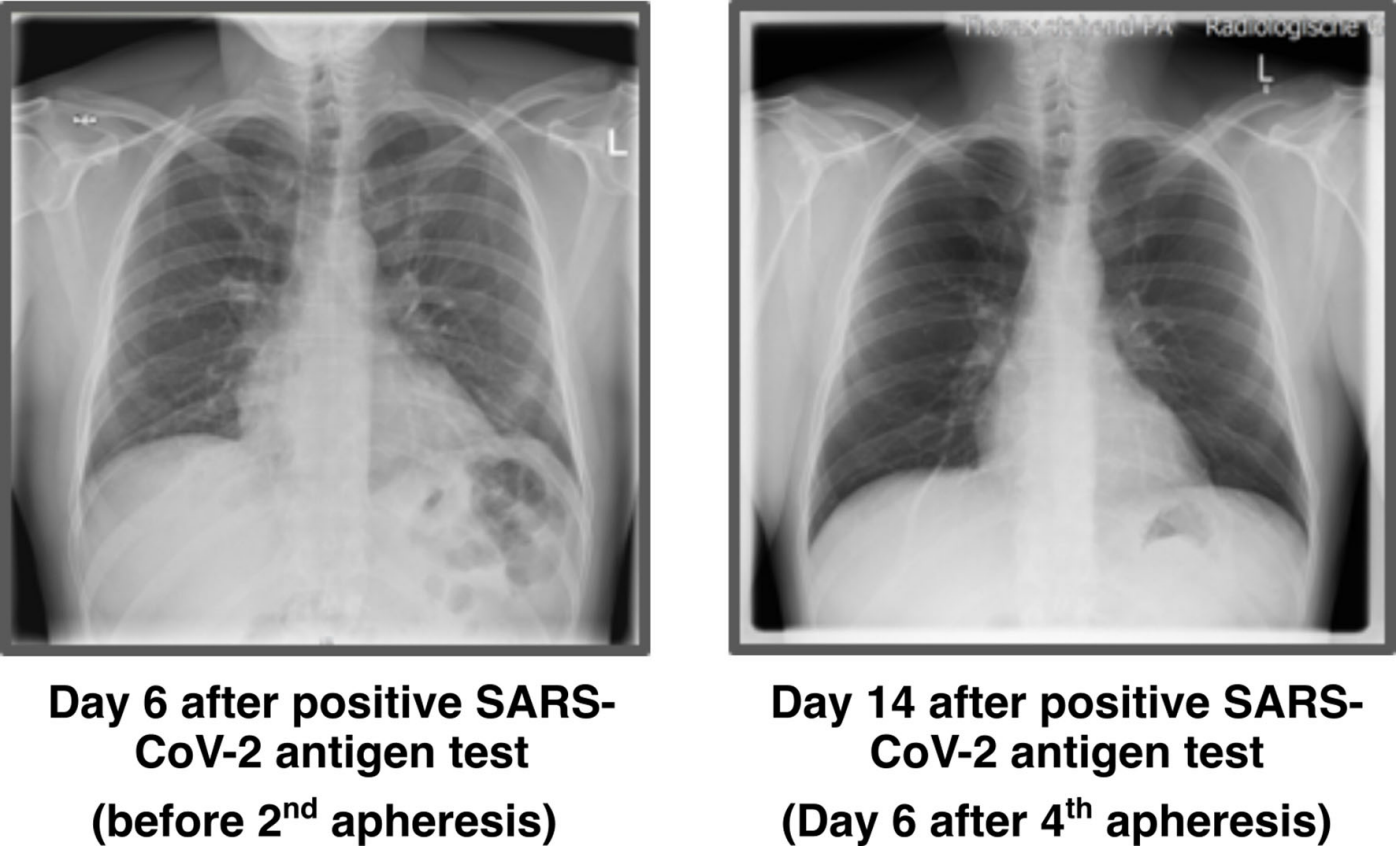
Chest X-ray images. X-ray thorax in 2 planes left adjacent. 6 days: Streaky compressions of the lung in the lower field/retrocardial left side. A low-grade concomitant effusion on the left. Heart normal size, no congestion. Upper mediastinum slender. No pneumothorax. Bony thorax intact. 14 days: No compressions and effusions.
